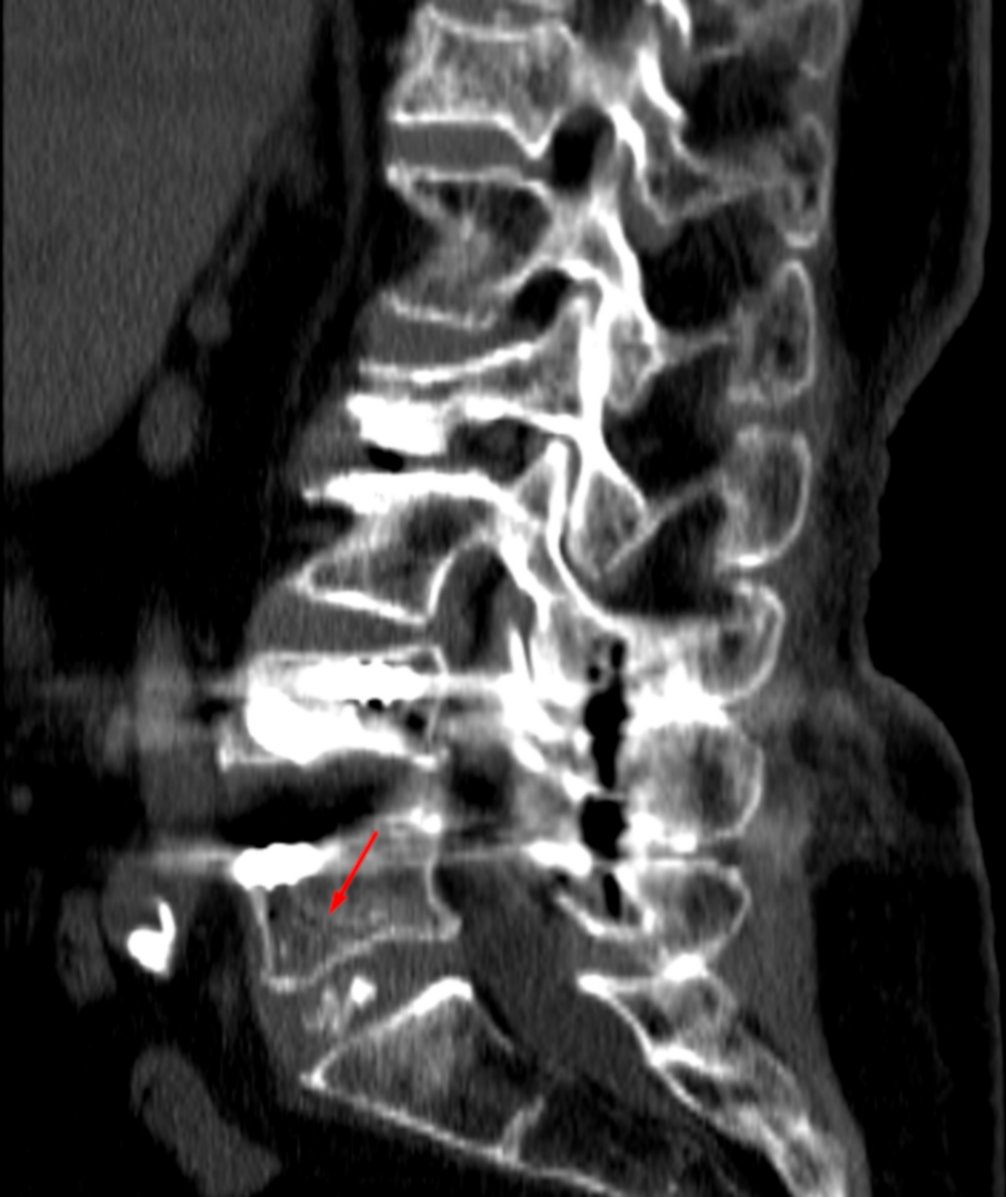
Sagittal CT Lumbar Spine Sagittal computed tomography (CT) lumbar spine revealing fracture line extending superior to and involving the inferior endplate of L5. Arrow indicates fracture.
